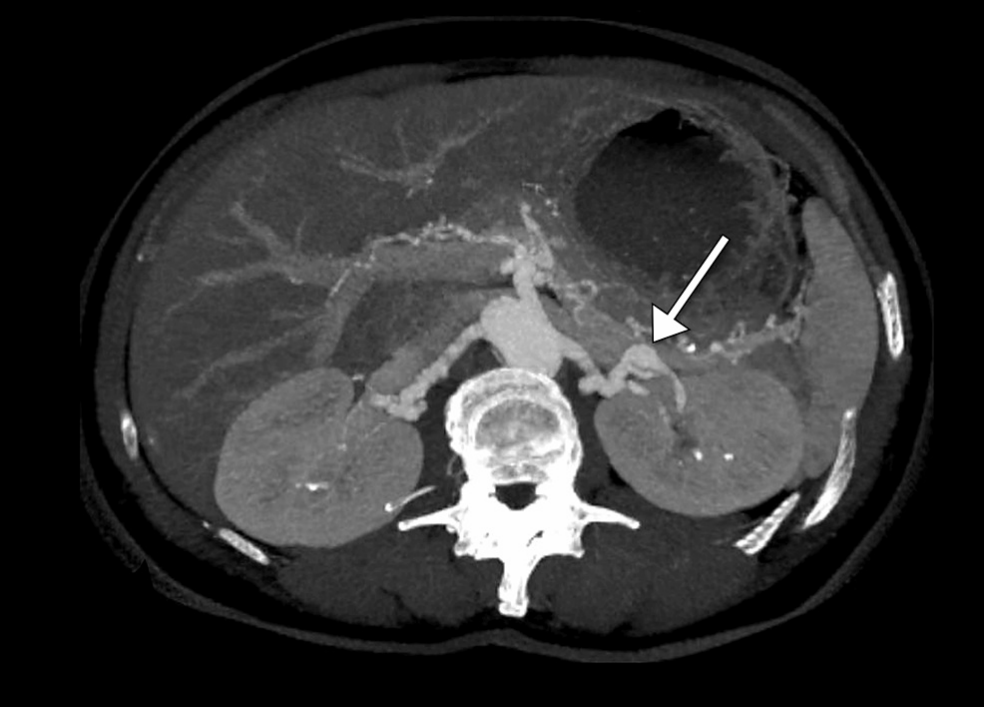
Axial CTA image of the abdomen showing left renal artery aneurysm (arrow) associated with a beaded appearance of renal and mesenteric arteries, consistent with fibromuscular dysplasia CTA: computed tomography angiography
